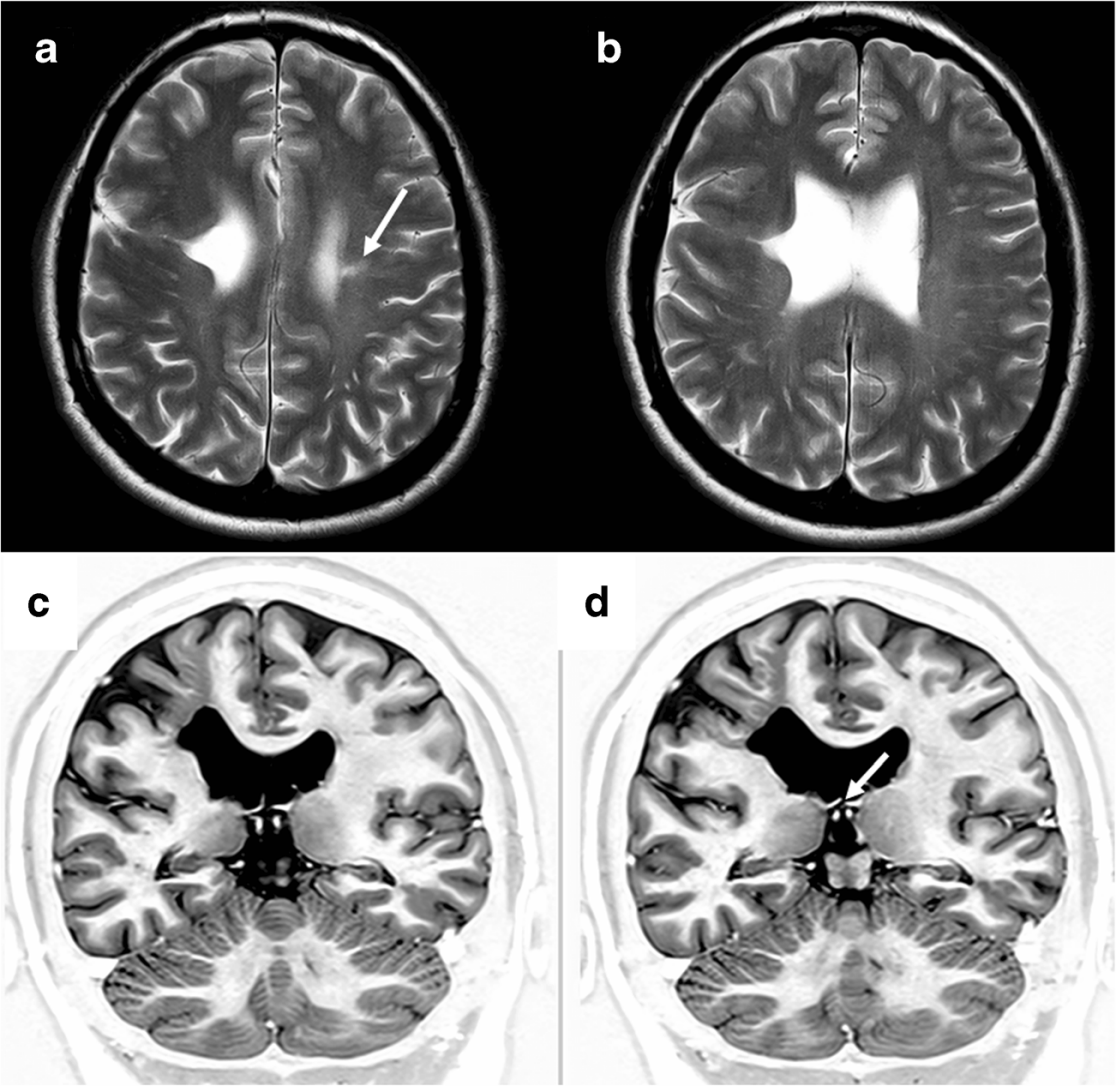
Unilateral schizencephaly (type 2). MR images of a 4-year-old child with focal epilepsy and developmental delay. Axial T2-weighted (a, b) and coronal inversion recovery (c, d) images show a CSF cleft with closely opposed borders lined with polymicrogyria. There is also a small area of gliosis in the white matter of the contralateral paracentral lobule (arrowed on a). The septum pellucidum is absent and the fornices lie abnormally low (arrowed on d). No other brain abnormalities were present
